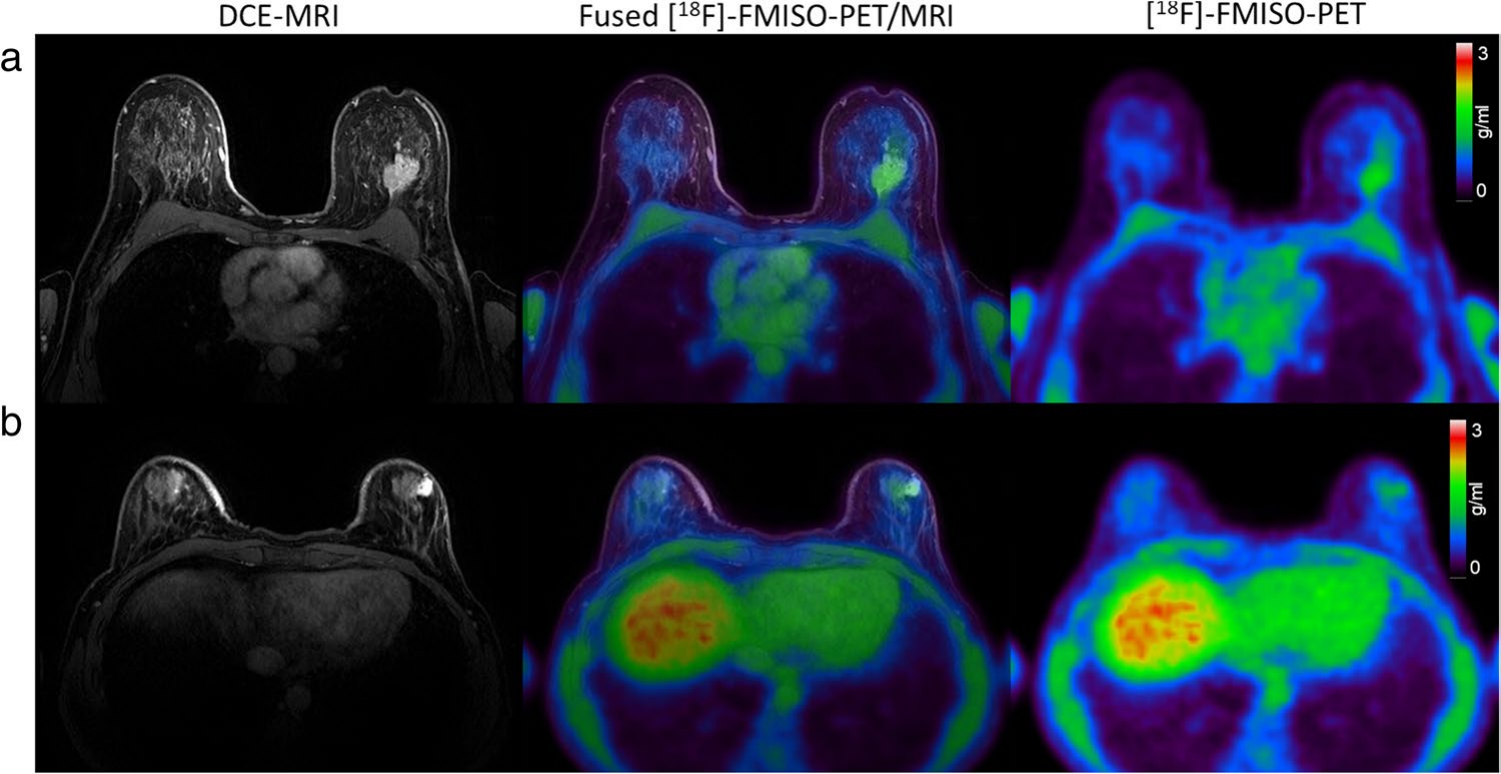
Representative transaxial images from two patients with ER + /HER2 − breast cancer. *Left*: Dynamic contrast-enhanced (DCE) MRI at peak enhancement; *centre*: [^18^F]-FMISO-PET (150–180 min p.i.) overlaid on the peak-enhancing DCE-MRI; *right*: [^18^F]-FMISO-PET (150–180 min p.i.). a Invasive ductal carcinoma (IDC), grade 2 with high [^18^F]-FMISO uptake in the tumour (SUV_max_ = 1.9; SUV_mean_ = 1.4; *T*_max_/*M* = 1.5). b Invasive mucinous carcinoma (IMC) with ductal carcinoma in situ (DCIS), grade 3, with moderate [^18^F]-FMISO uptake in the tumour (SUV_max_ = 1.2; SUV_mean_ = 1.0; *T*_max_/*M* = 1.0)
